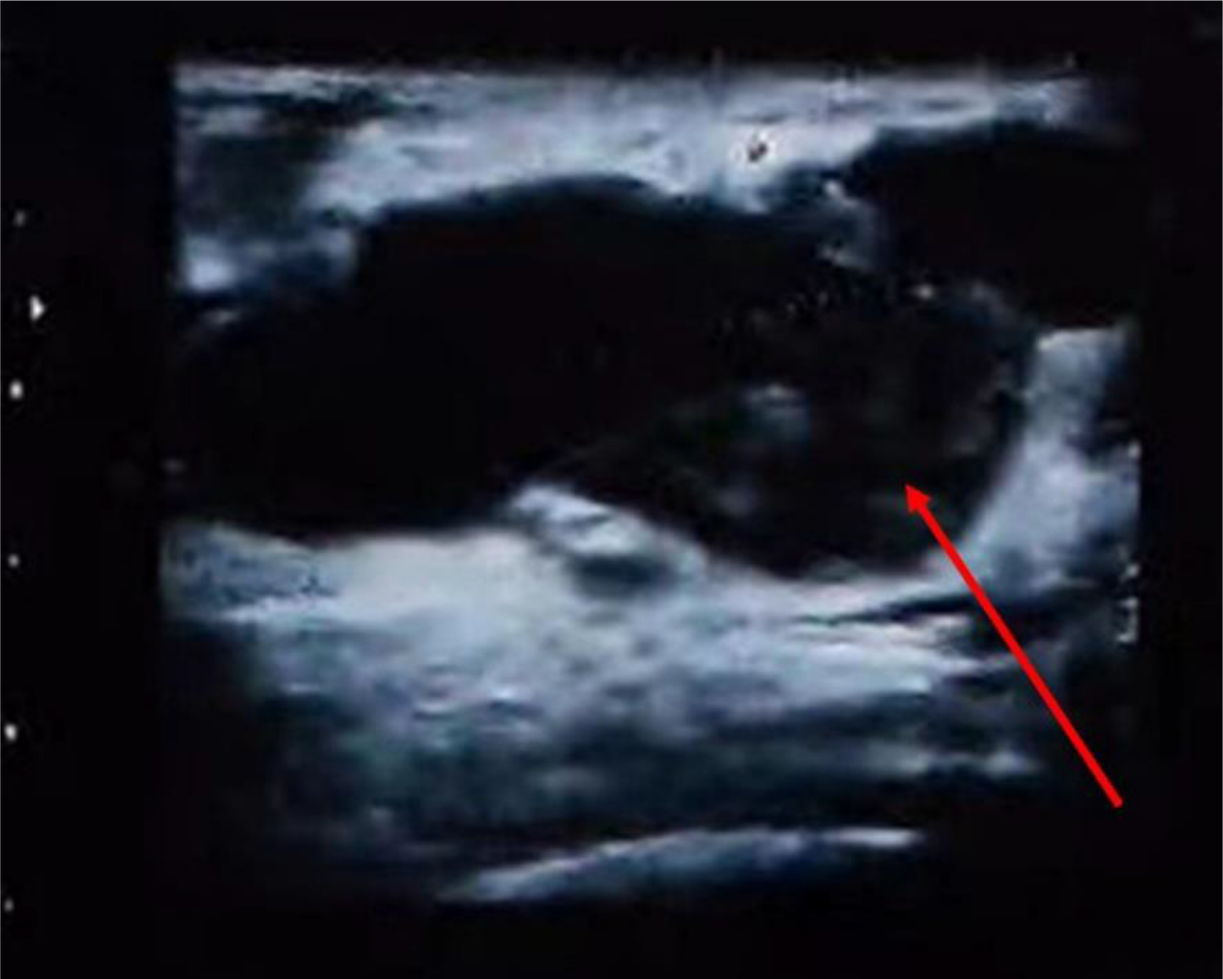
Breast color Doppler ultrasound of the patient at the first admission. The red arrow shows the tumor.
